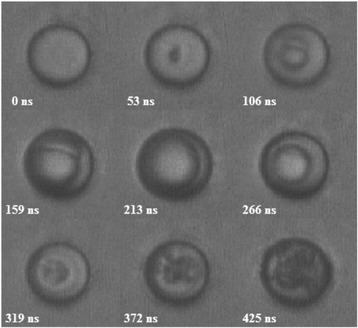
Consecutive images of ADV at 15 °C superheat produced by ultrasound at frequency of 3.5 MHz and pressure of 4.5 MPa with the initial droplet radius of 5.4 μm, with courtesy of [76]
